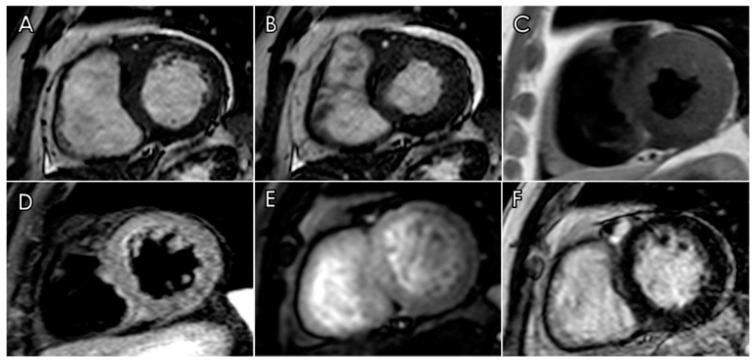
CMR in patient with coronary IgG4-RD. Short axis images performed along the basal slice showing the peri-coronary pseudotumor encasing LAD during end-diastole (A) and end-systole (B). The lesion appeared isointense to myocardium in balanced steady-state free precession (bSSFP) sequence (A,B), hypointense in T1-weighted (C), and T2-weighted sequences with short-tau inversion recovery (STIR) (D). The pseudo-mass did not show any early enhancement during the arterial first-pass (E), but late gadolinium enhancement (LGE) was seen in the T1-weighed phase sensitive inversion recovery (PSIR) sequence (F).
